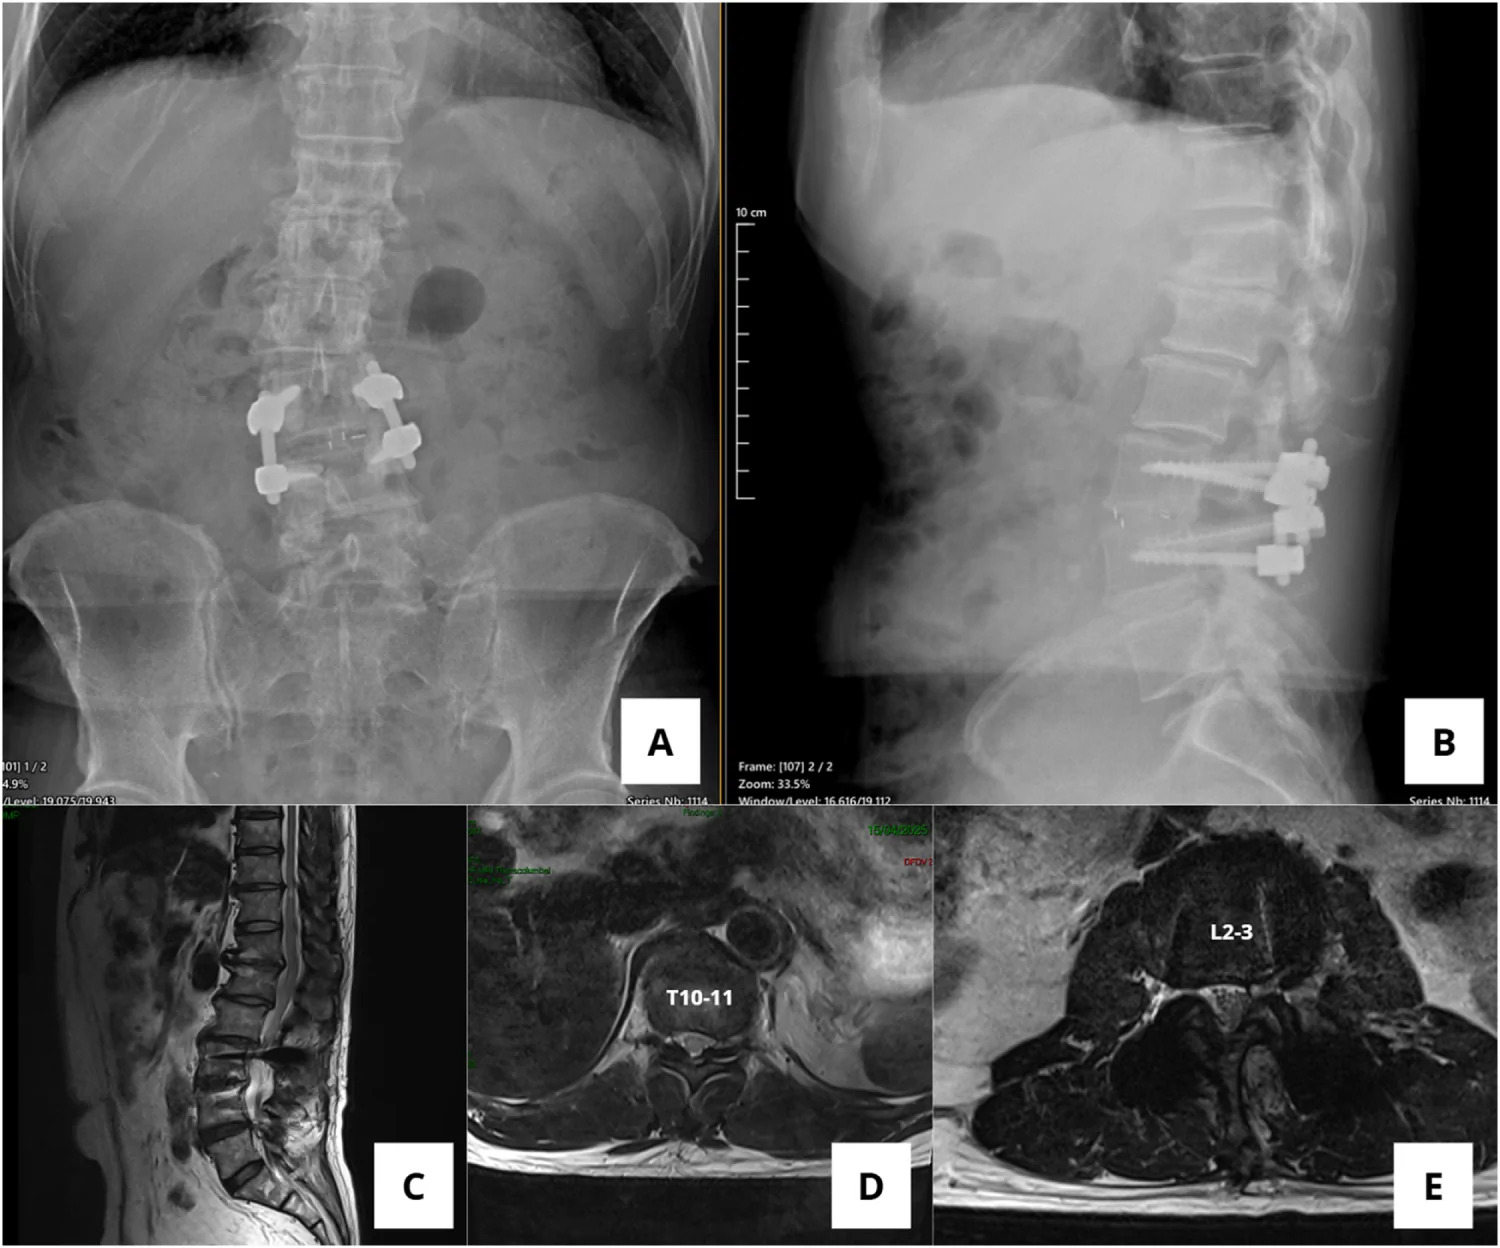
Preoperative imaging showing (A and B) X-ray AP and lateral view demonstrated pedicle screw and rod fixation at L3 and L4 with interbody fusion from a previous operation. Preoperative MRI T2-weighted images showing (C) spondylolisthesis at L2–L3 Meyerding Grade 1 and degenerative disc disease at L2–L3. (D) Axial view of T10–T11 showed spinal canal stenosis at T10–T11 Schizas grade B. (E) Axial view of L2–L3 showed spinal canal stenosis at T10–T11 Schizas grade B.
